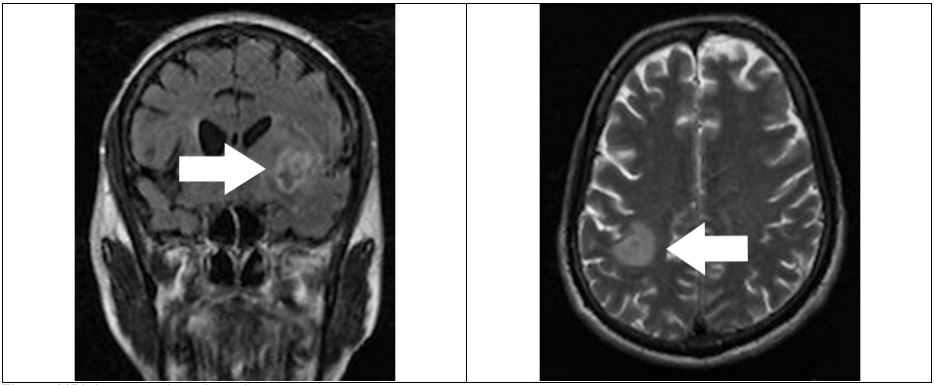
MRI demonstrating tuberculoma. *MRI*, magnetic resonance imaging
